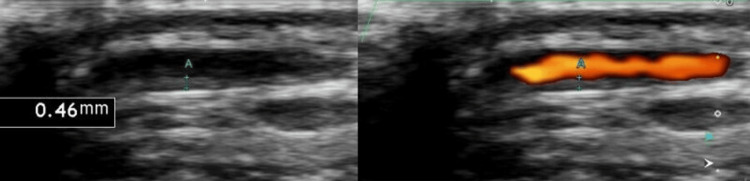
Ultrasonography of the right temporal artery. Ultrasonography of the right temporal artery revealing vessel wall thickening (0.46 mm) and luminal narrowing with power Doppler mode on the right image.
